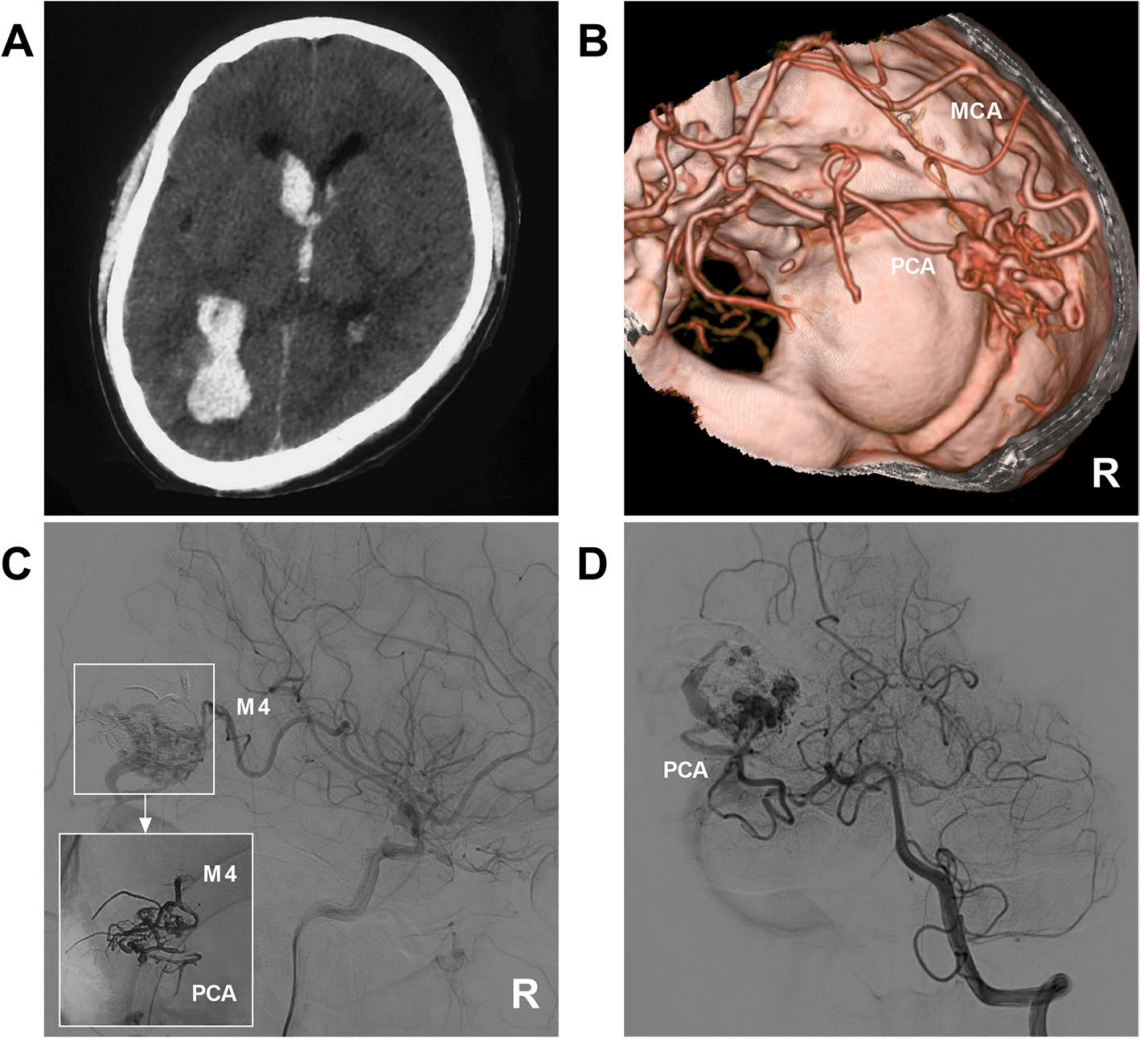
EVT of a type IIIb MCA-BAVM. A CT showed hemorrhage in the right parietal and occipital lobes extending into the ventricle. B CTA showing that the BAVM was supplied by the M4 segment of the MCA and PCA. C Right ICA angiogram showing that the BAVM was partially embolized via the MCA; the picture in the picture shows the casting Onyx. D Vertebral artery angiogram showing that the PCA still supplied the BAVM, but the volume of the BAVM nidus was reduced. Abbreviations: BAVM: brain arteriovenous malformation, CT: computed tomography, CTA: CT angiography, EVT: endovascular treatment, ICA: internal carotid artery, MCA: middle cerebral artery, M4: fourth segment of the MCA, PCA: posterior cerebral artery, R: right
